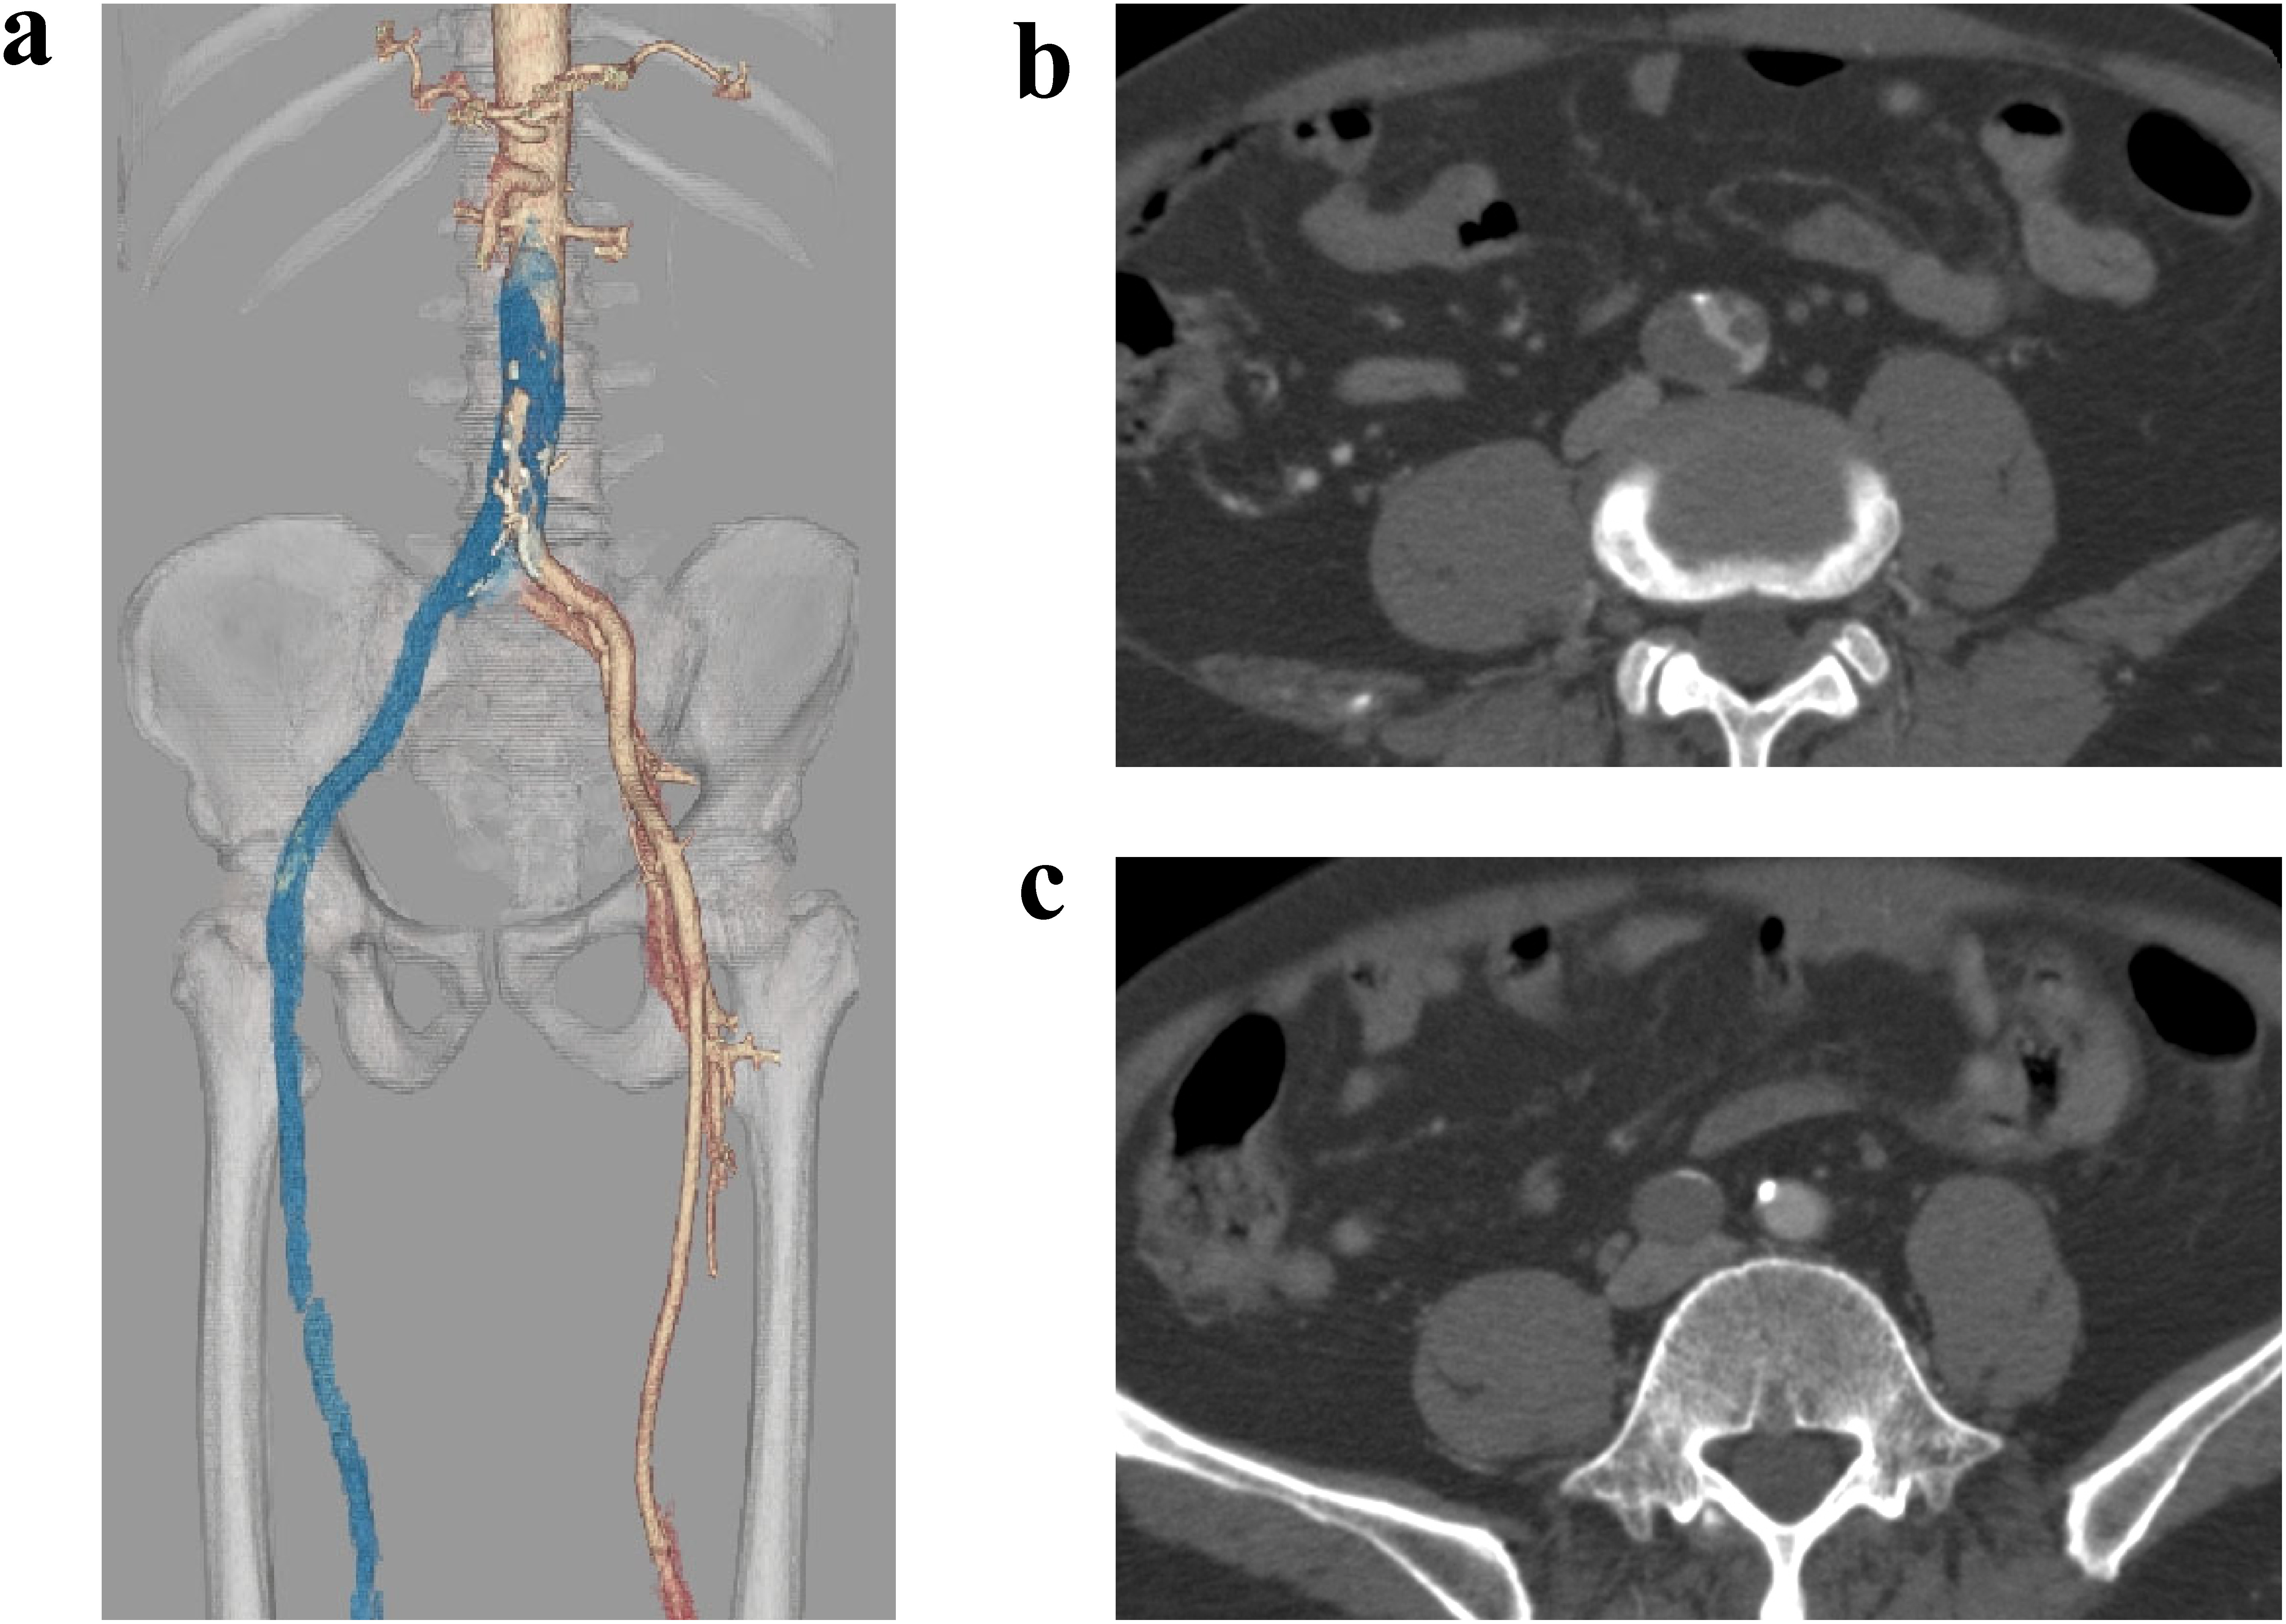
Fig. 2 Three-demensional reconstruction of computed tomography angiography demonstrated occlusion of the right common iliac artery, which was colored light blue (a). There was a stenosis because of thrombosis in the infrarenal abdominal aorta (b). The right common iliac artery with no contrast in the vessels distal to the occlusion (c).
